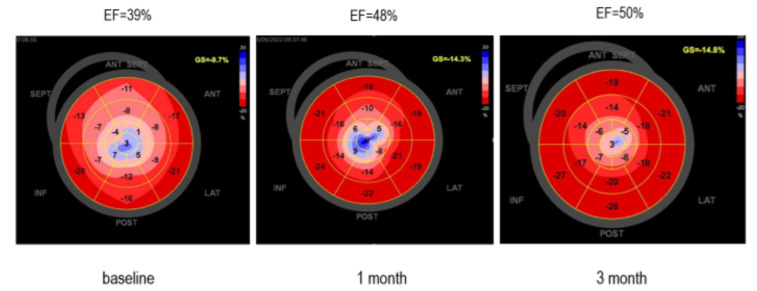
During the follow-up, echocardiography exams were performed and the progression of LVEF values from 38 to 50% as well as GLS from −9.7% to −14.8% was observed. The blue-colored zones in the bull’s eye plot estimated by strain were improved. The type of SCAD and TIMI flow grade may be correlated with myocardial infarct size [4]. About 26% of SCAD patients have a very small reduction in LVEF < 50% and over 50% have subsequent normalization of wall motion abnormality and LVEF on follow-up assessment [4]. Different pathophysiology of the SCAD ST segment elevation of myocardial infarction (STEMI) compared with Type 1 STEMI might be an explanation of the differences in infarct size and in infarct zone appearance. In SCAD, the forming of infarct size could be a balanced process. OCT studies showed that the absence of a fenestration in the vessel wall leads to increased false lumen pressure and compression of the true lumen, resulting in larger infarct size [5]. A hematoma which can compromise the true lumen without obstruction is often found. Furthermore, the absence of a conditioning effect and collateralization induced by a prior-fixed stenosis (as found in Type 1 STEMI) might tend to increase infarct size in the event of a SCAD-STEMI [6]. SCAD survivors with apparent endocardial sparing differ from STEMI patients with a typical pattern of endocardial involvement [6].
